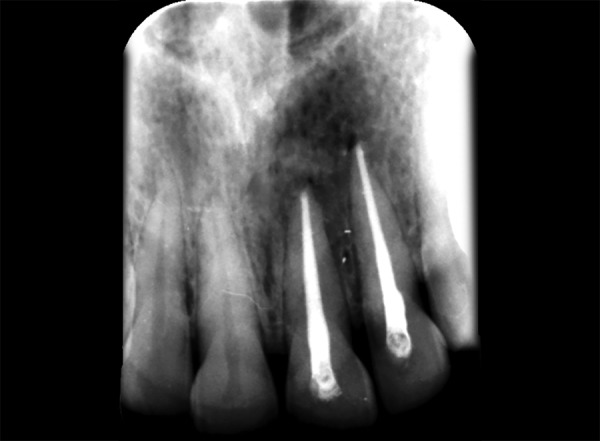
Eighteen months postoperative radiograph
